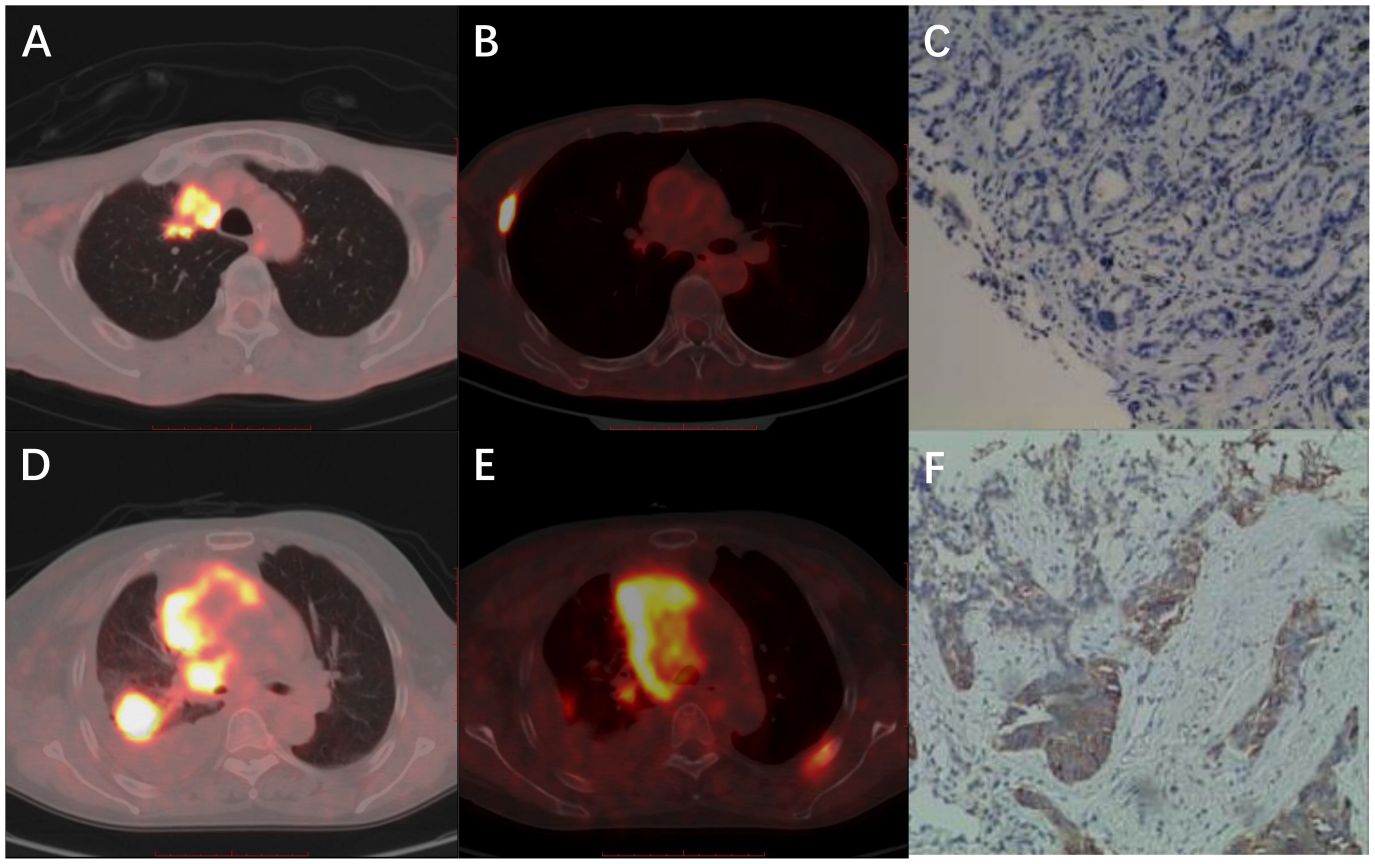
Representative images of PET/CT and IHC. (A-C) A 68-year-old man with ADC. The biopsy site was from rib metastasis and PD-L1 expression was negative. The SUVmax, SUR-L, and SUR-BP of primary tumors were 15.72, 6.89, and 9.14 and of rib metastasis were 19.92, 8.74, and 11.58. (D-F) A 66-year-old woman with SCC. The biopsy site was from lung primary tumors and PD-L1 expression was positive. The SUVmax, SUR-L, and SUR-BP of primary tumors were 14.76, 5.35, and 10.32, and those of lung primary tumors were 5.23, 1.89, and 3.66, respectively.
